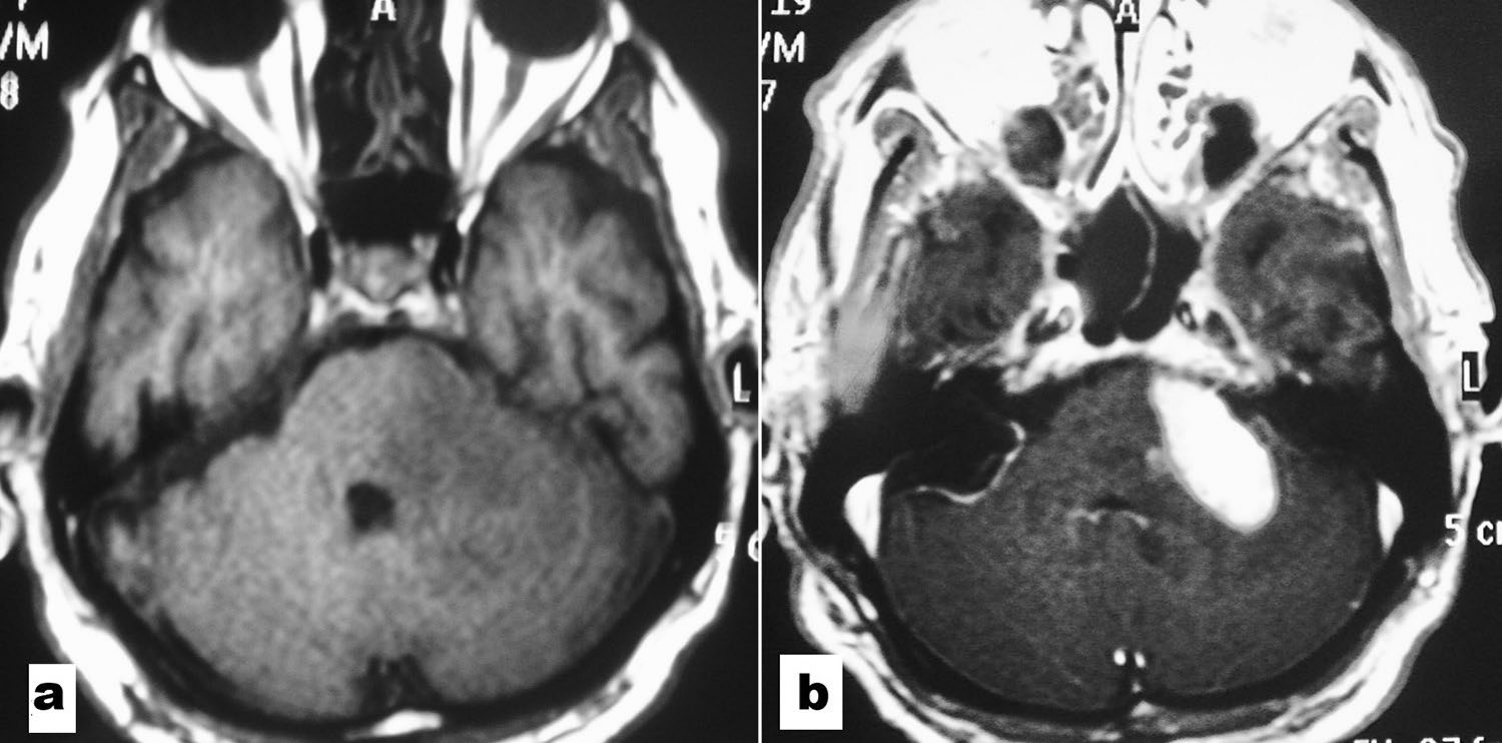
Magnetic resonance imaging appearance of an atypical brainstem tumor. (a) T1-weighted axial image without and (b) with gadolinium demonstrates an enhanced prominent contrast and lesion outside the limits of the pons.
